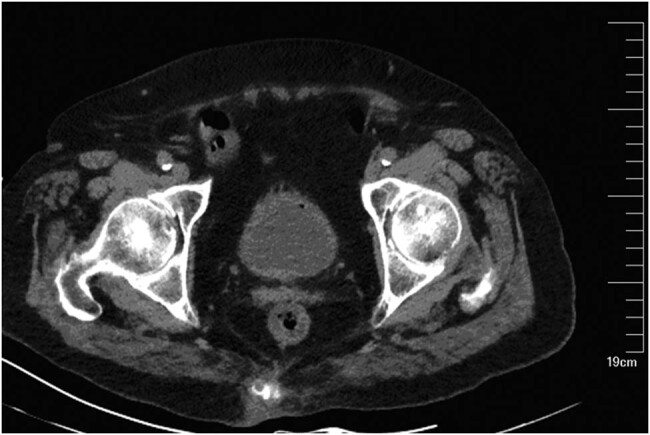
An abdominal CT scan showed thickening bladder wall and a little gas in the bladder.
